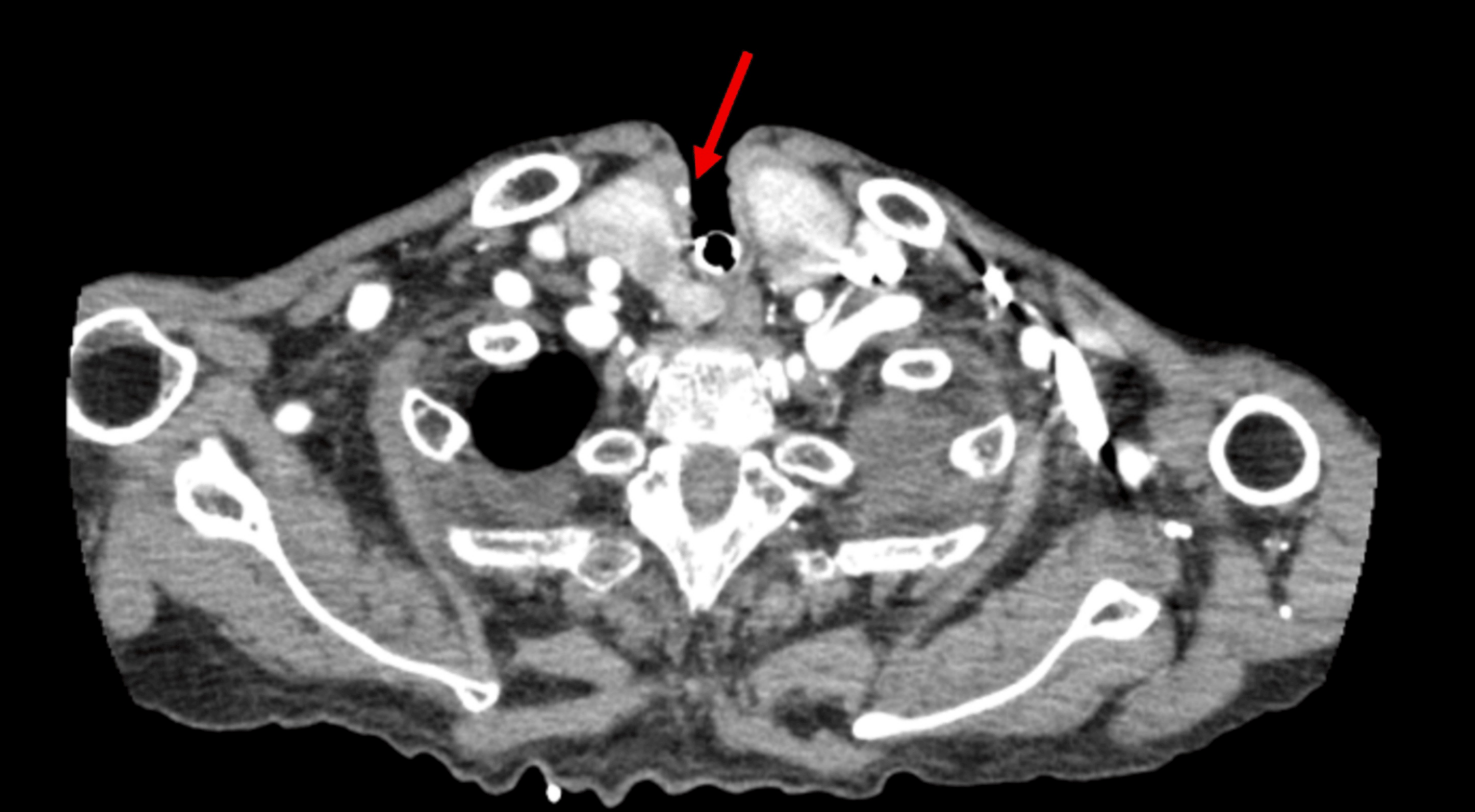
Contrast-enhanced CT showing a superior thyroid artery pseudoaneurysm after the first bleeding episode Axial arterial-phase contrast-enhanced CT performed after the first massive bleeding episode shows a focal enhancing lesion adjacent to the tracheostomy stoma, compatible with a pseudoaneurysm of the right superior thyroid artery (arrow).
